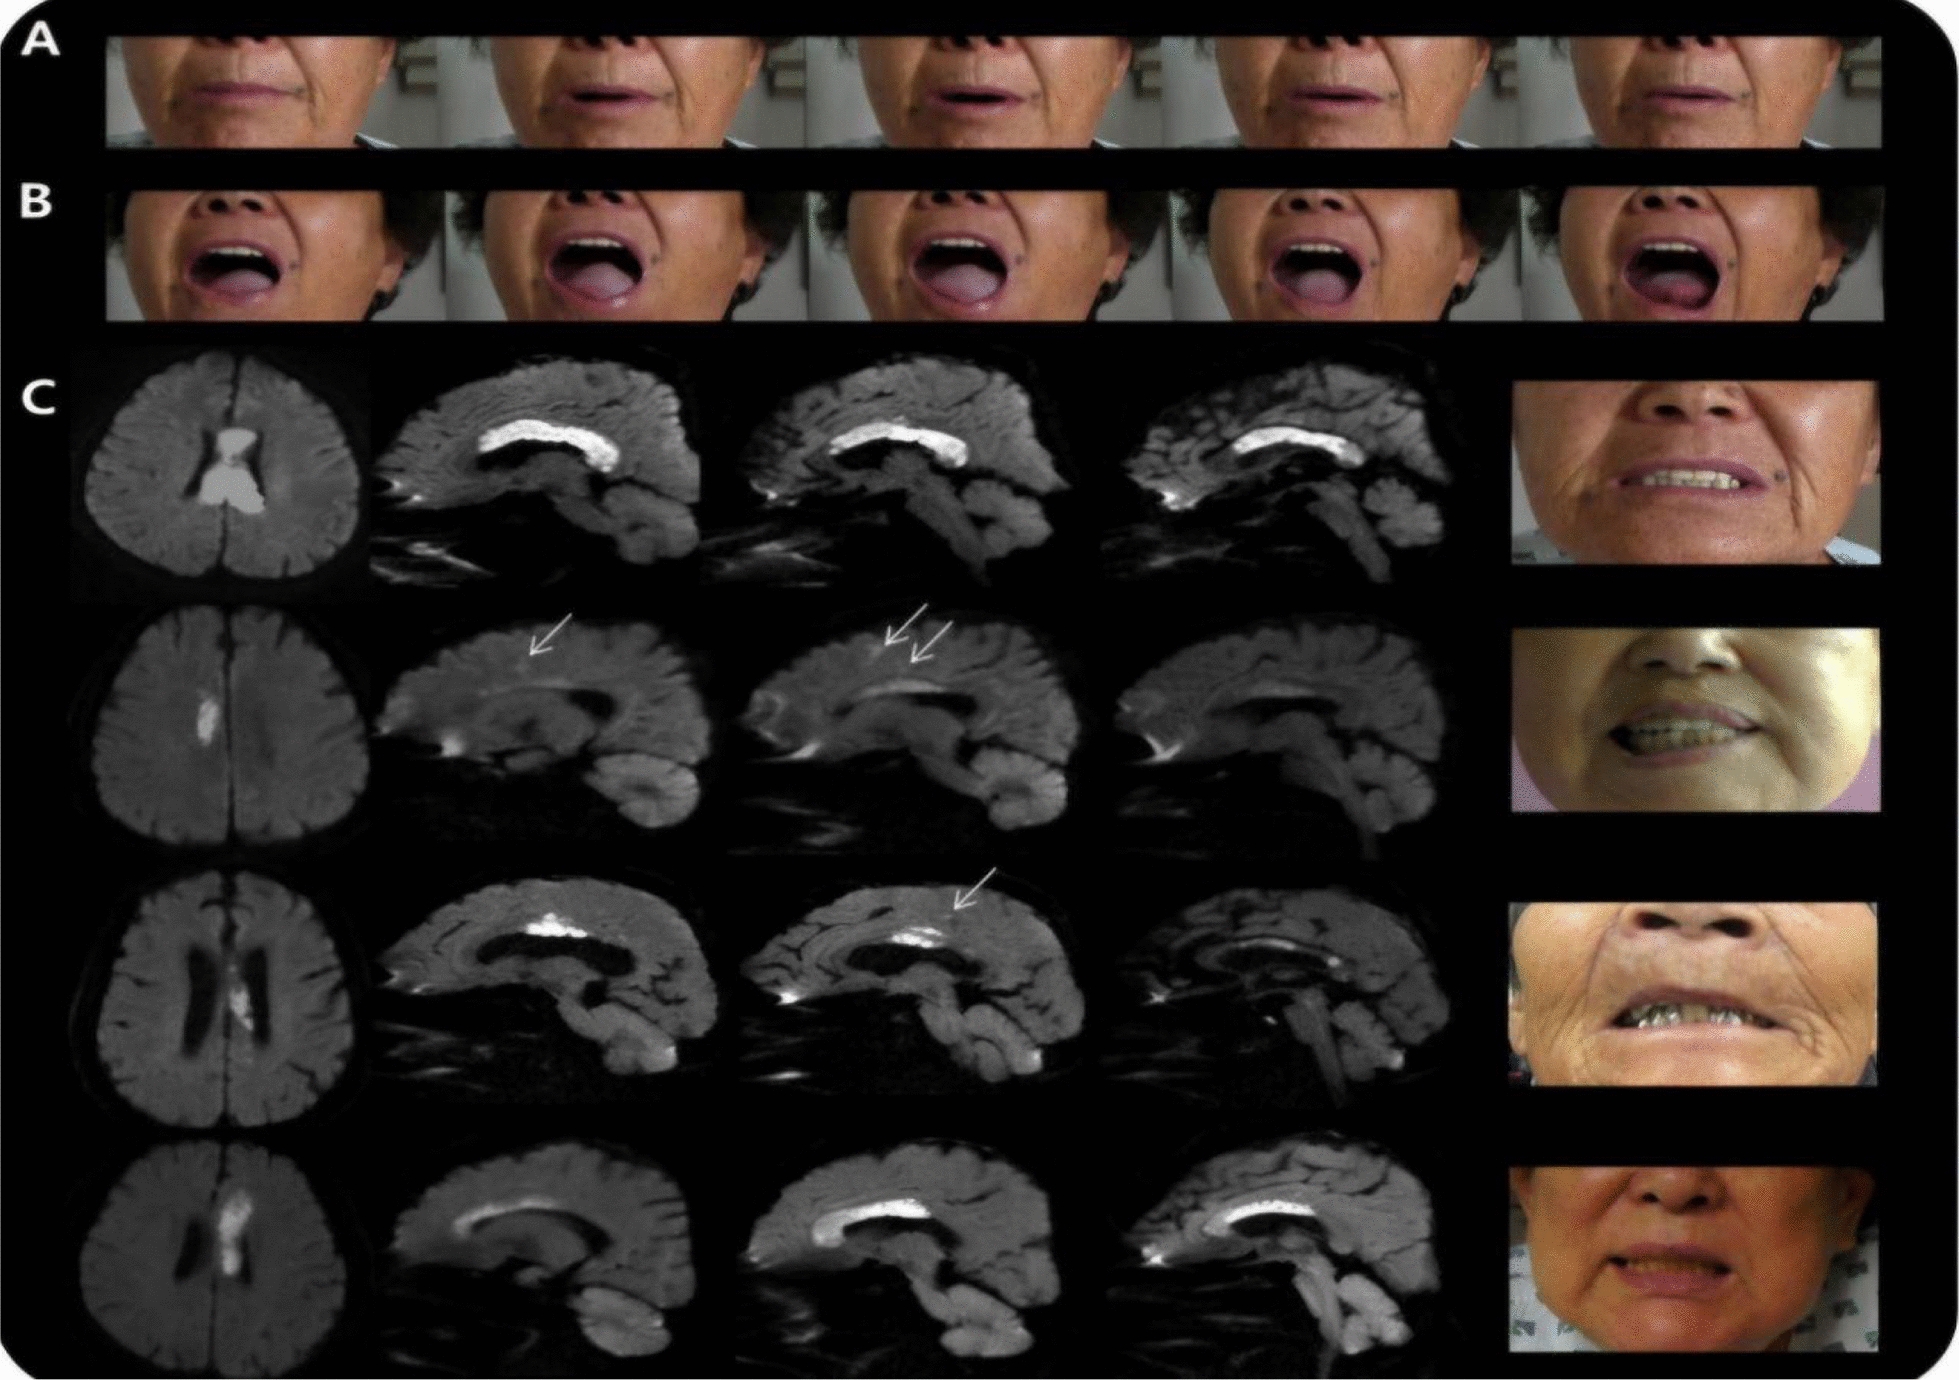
Facial-labial movement asymmetry in callosal AHS: sequential clinical photos (A–C) with corresponding diffusion MRI lesions (arrows) [56]
